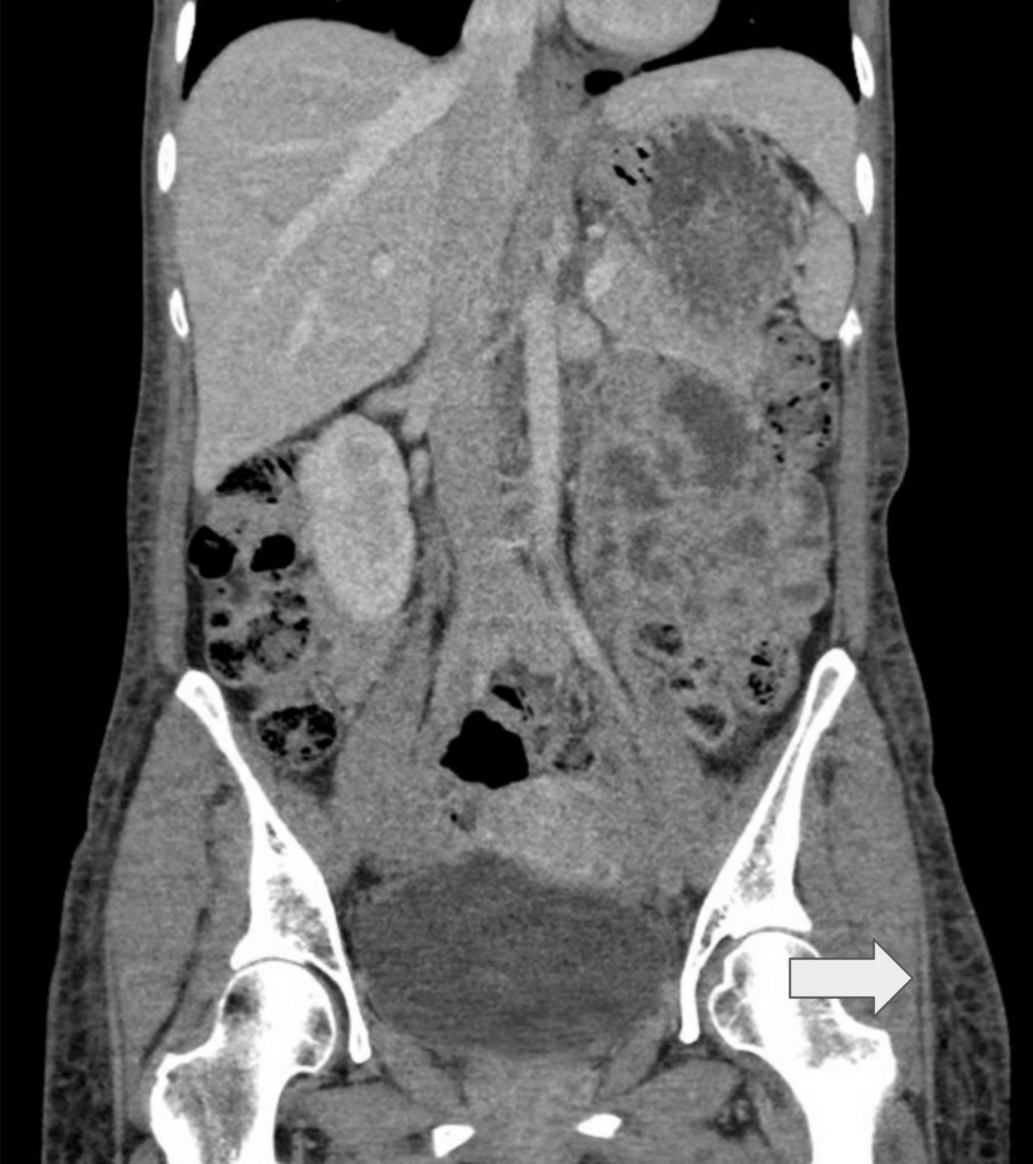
Contrasted computed tomography scan of the abdomen and pelvis A bilateral small amount of pleural effusions, diffuse subcutaneous edema, and mild interlobular septal thickening in the visualized lung bases may be related to fluid overload. The arrow points to subcutaneous edema.
